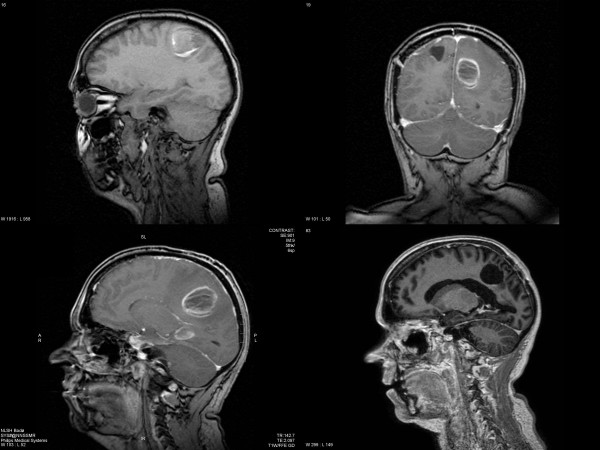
Magnetic resonance imaging scans of the brain. Upper left: the first lesion that led to diagnosis of metastatic melanoma. Lower left and upper right: 3 months after resection of the first lesion, 2 new metastases were diagnosed. Lower right: status 27 months after first diagnosis with residual changes after 2 radiosurgery treatments in the temporal lobe and a resection cavity in the parietal lobe.
